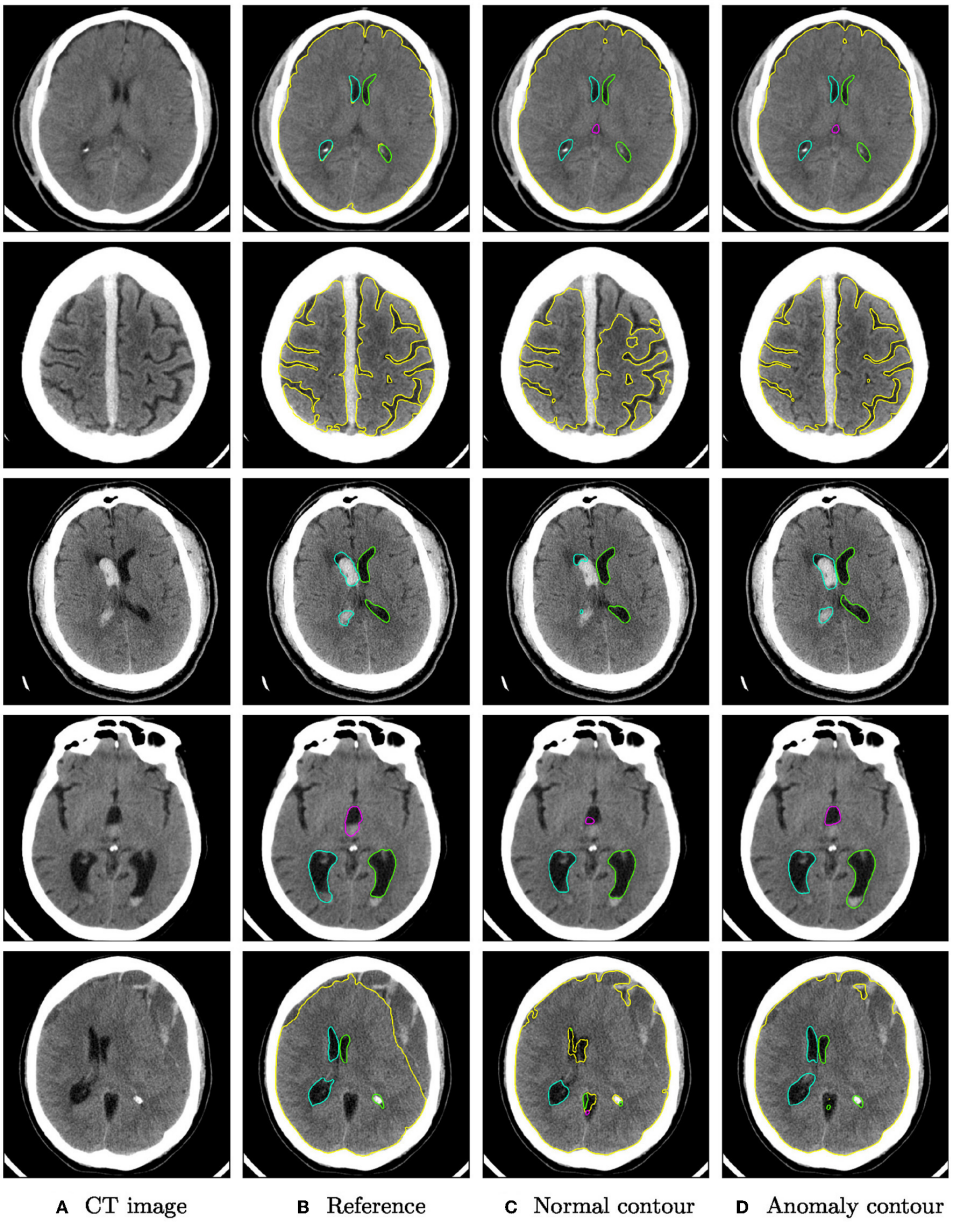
Qualitative segmentation examples from the RSNA dataset of parenchyma (yellow) and ventricles (left ventricle: green, right ventricle: cyan, third ventricle: magenta, fourth ventricle: purple). Each row shows from left to right (A) the original CT slices, (B) the reference segmentation, (C) the segmentation from the algorithm trained on normal cases only, and (D) the segmentation from the algorithm trained on normal cases and hemorrhage cases. For better visibility, not all structures are shown for all cases.
